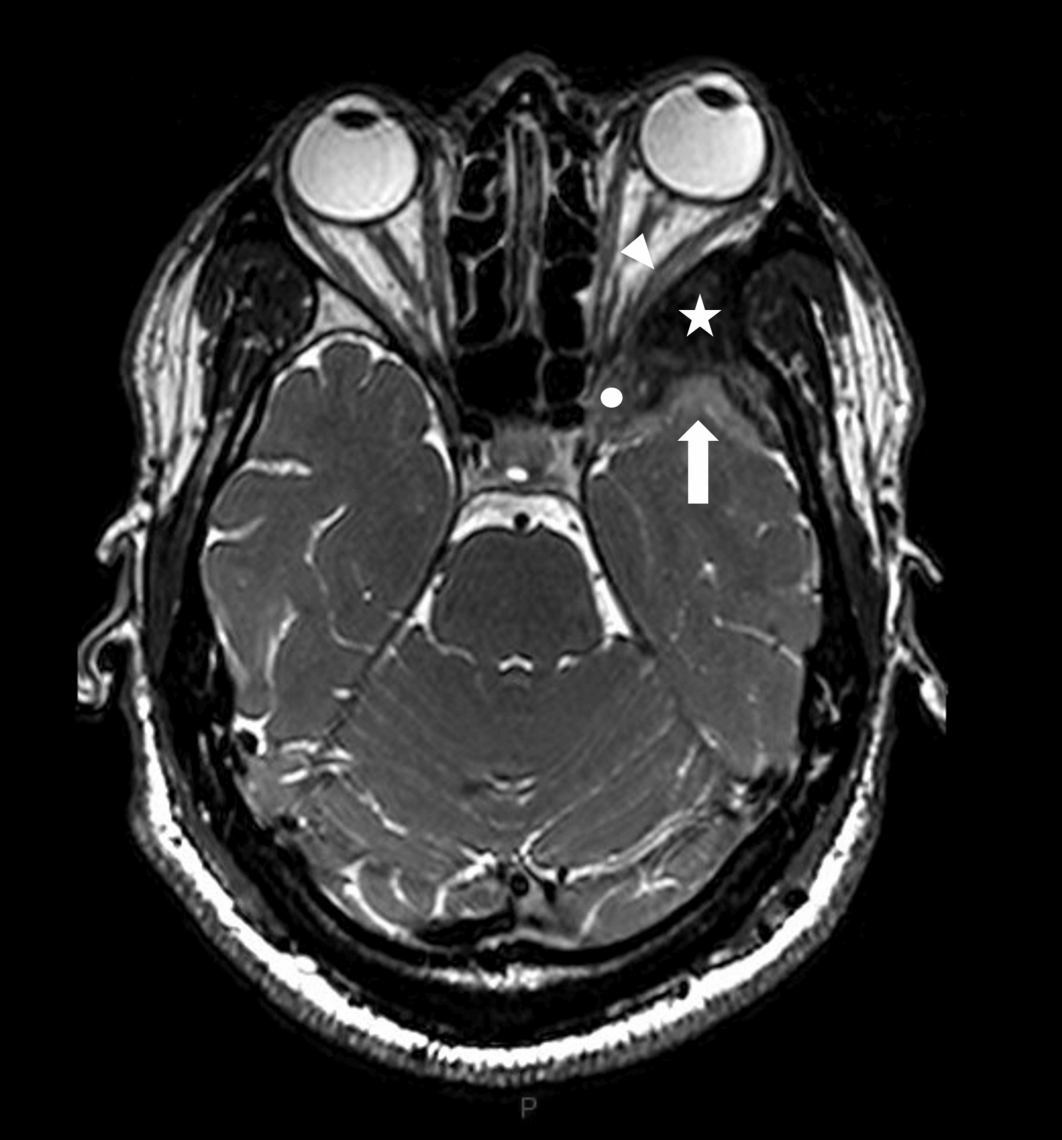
Preoperative axial T2-weighted brain MRI. The lesion is centered on the left greater wing of the sphenoid bone (white star), with associated meningeal thickening at the temporal apex (thick arrow), involvement of the orbital canal (white circle), extension into the orbit (arrowhead), and the anterior portion of the cavernous sinus.
